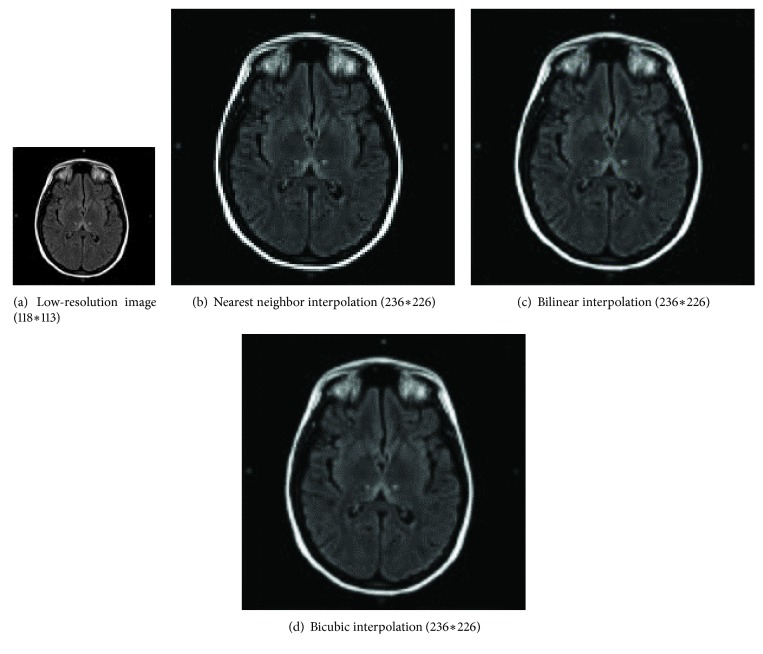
Three conventional SRR methods (MRI: brain).
